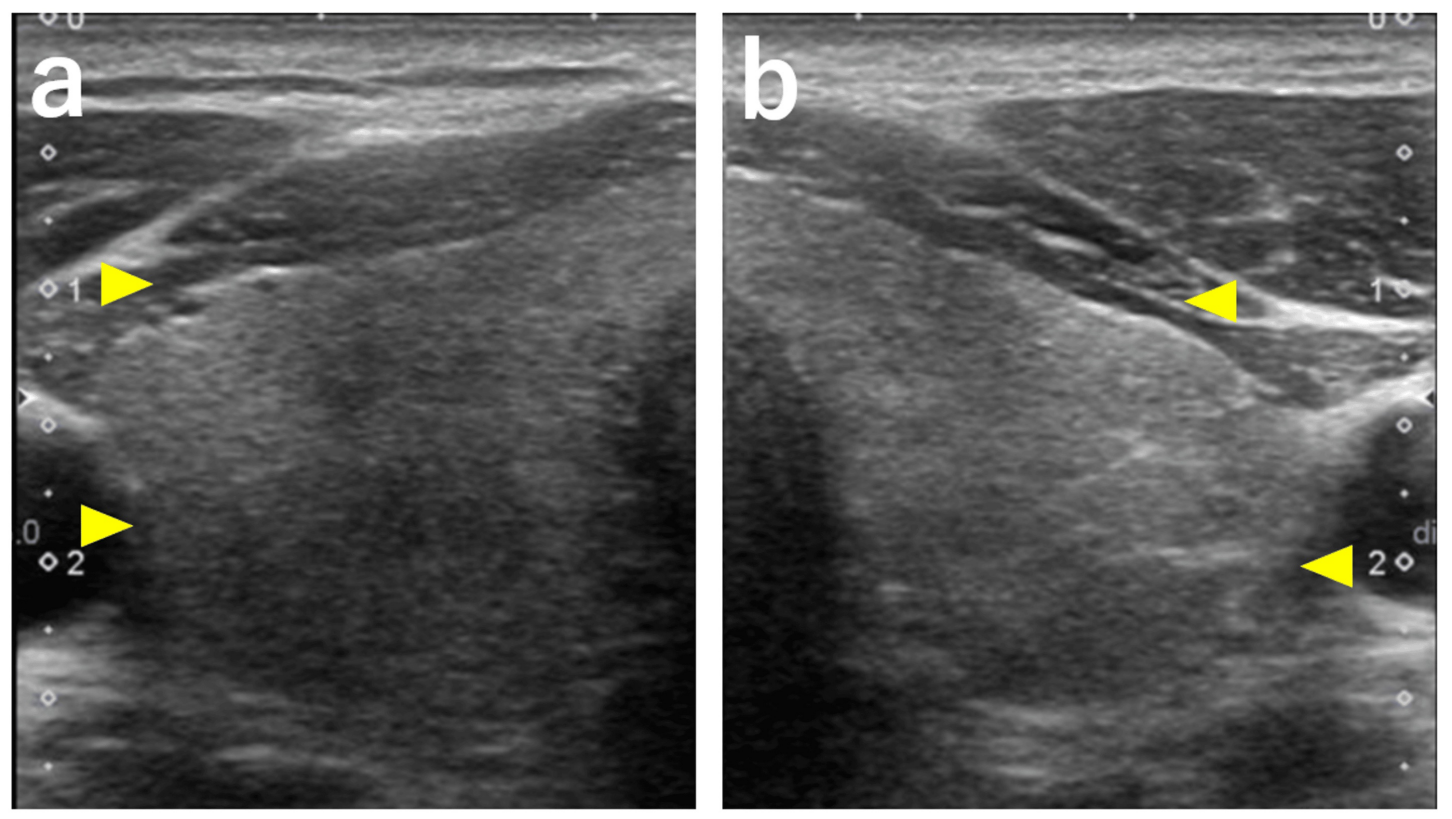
Thyroid ultrasonography (a) Right lobe and (b) Left lobe Mild thyroid enlargement with parenchymal coarseness, but no increased vascular flow was observed (arrowhead).
